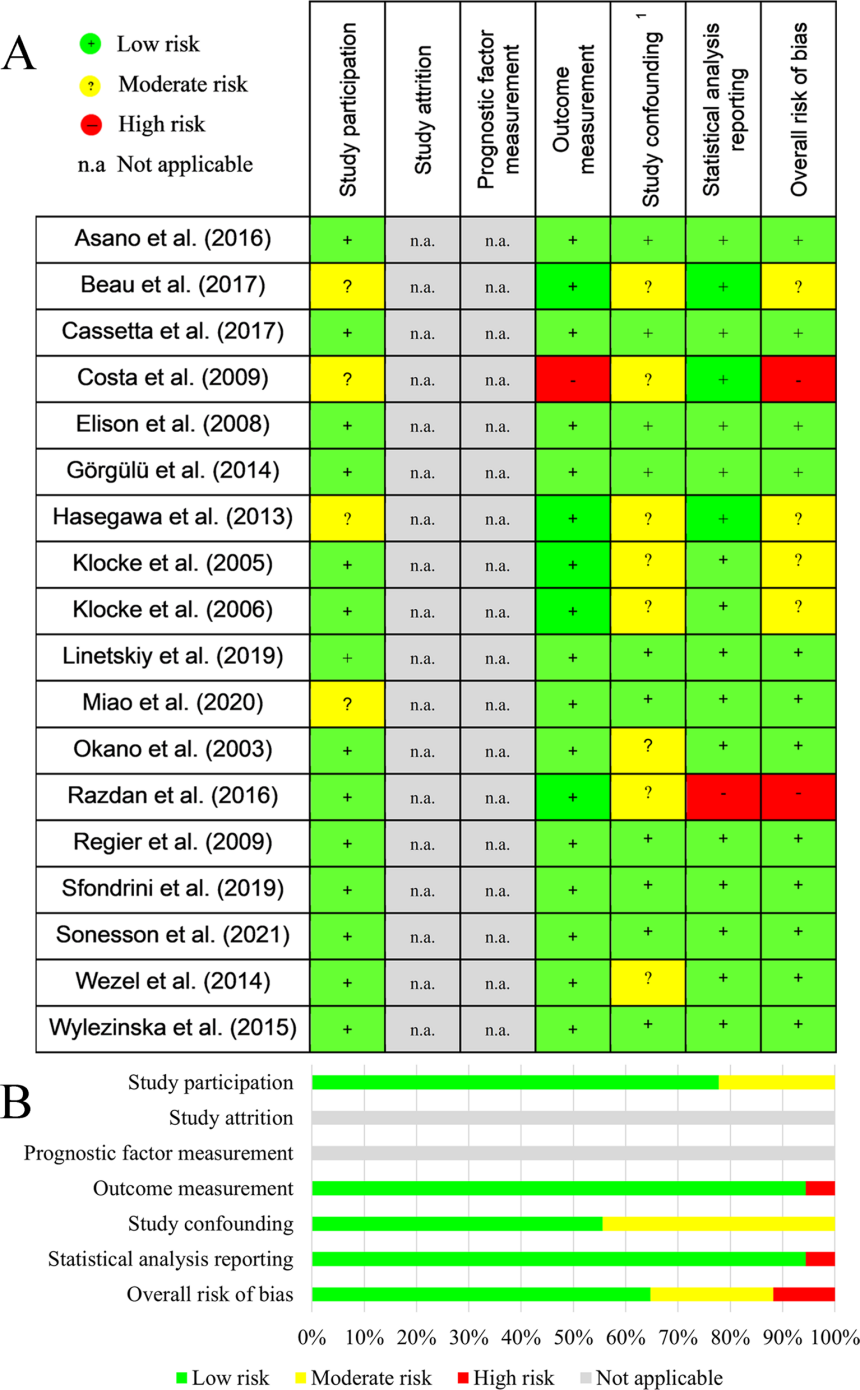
Risk of bias assessment on study level (A) and across studies (B). 1: Assessed confounding factors are dental materials, MRI sequences, magnetic force
